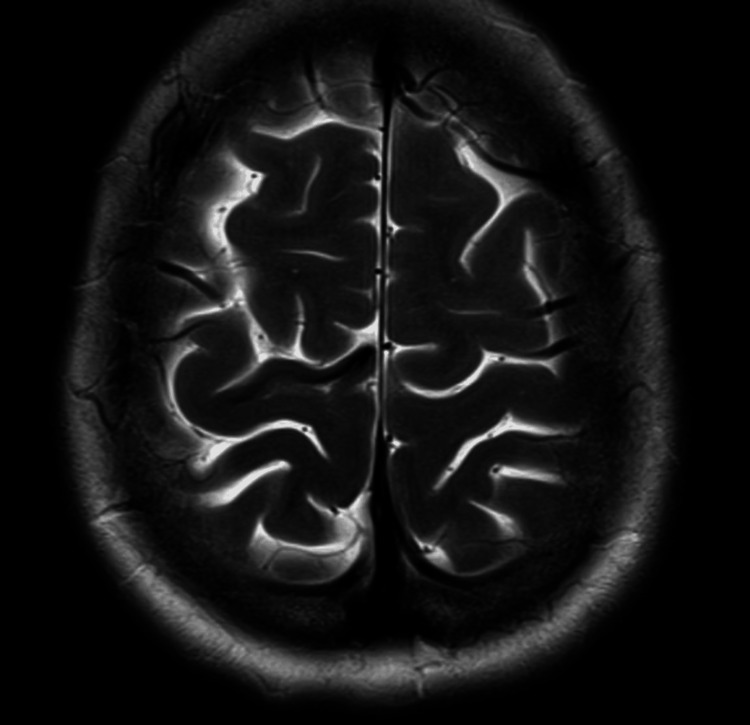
T2 axial MRI showing resolution of haemorrhage, two months post admission
